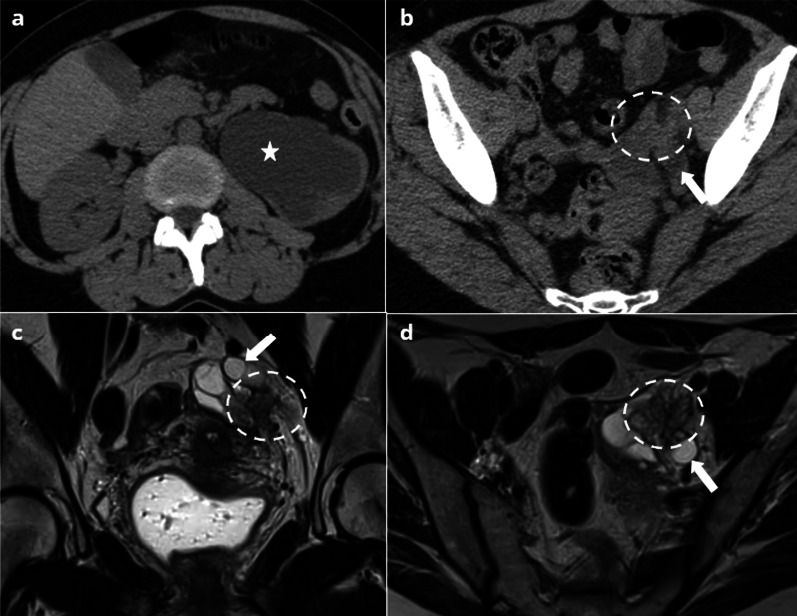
41-year-old female presenting with acute left lumbar region pain. No history of endometriosis. CT (a, b) shows a left hydronephrosis (star) and dilated ureter (arrow), up to a retractile mass located at the level of the ovarian fossa (dotted circle). T2W MRI (c, d) shows a stellar periureteral mass (dotted circle) responsible for obstruction (arrow). Nephrectomy was performed because of the complete loss of left kidney function, confirming the diagnosis of left ovarian and parametrial endometriosis, responsible for an extrinsic obstruction of the ureter
